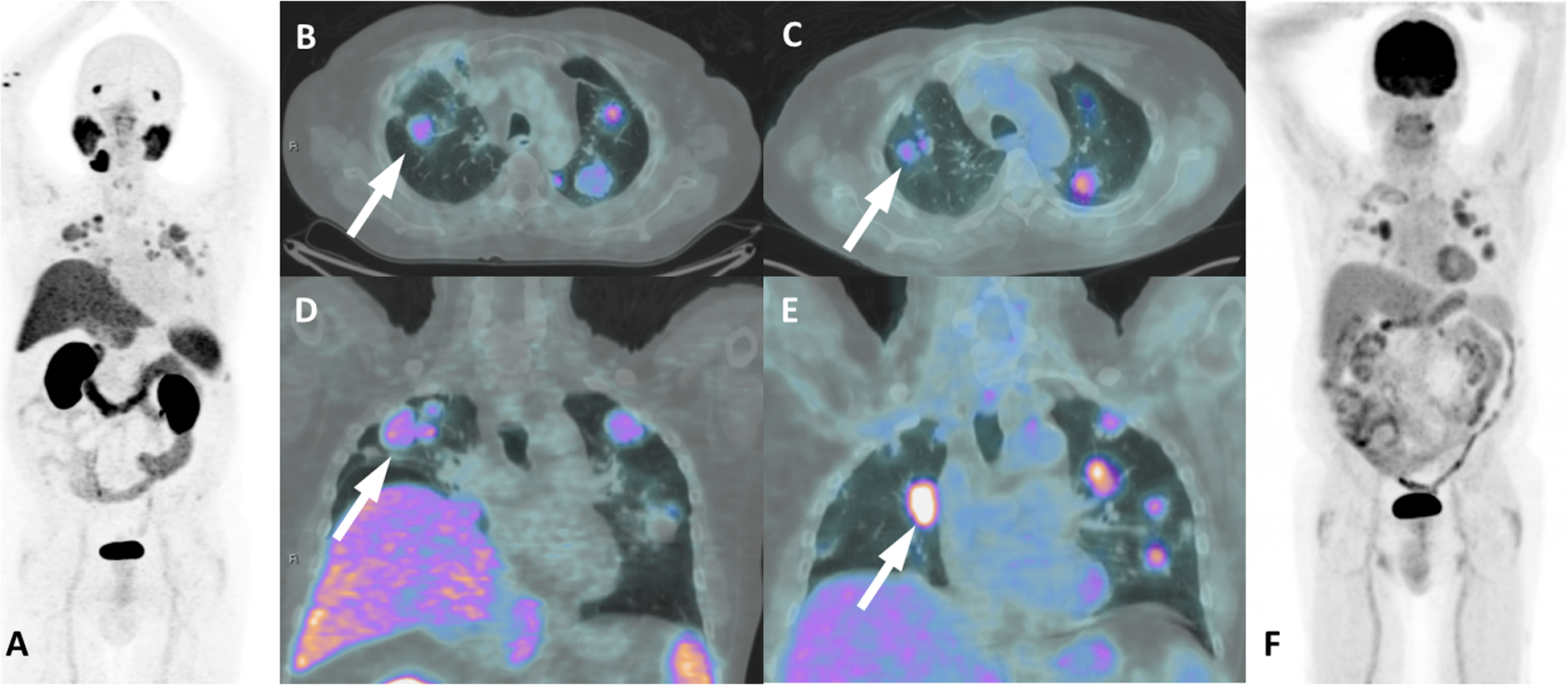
Metastatic adenoid cystic carcinoma in ^68^Ga-PSMA and ^18^F-FDG-PET/CT. ^68^Ga-PSMA-PET/CT MIP (a), axial and coronal PET/CT (b, d) and ^18^F-FDG-PET/CT MIP (f), axial and coronal PET/CT (c, e) images show multiple solid nodules (arrows) with a heterogeneous moderate to intense uptake of both tracers. This patient was referred to treatment with Lu-PSMA after progressive disease despite all previous treatments
